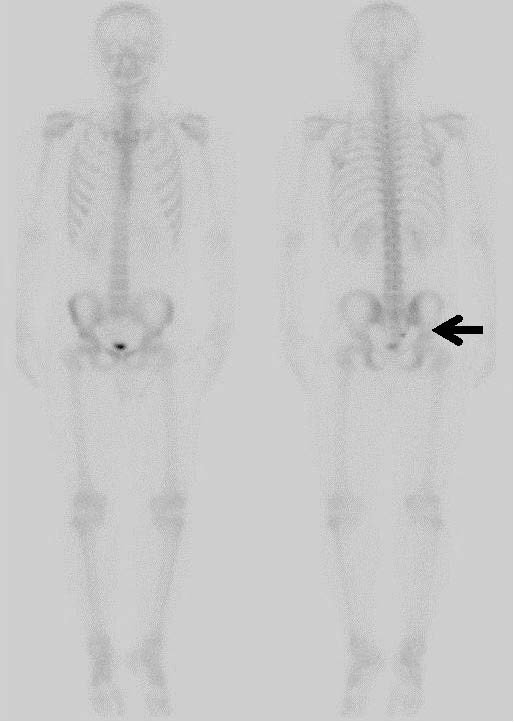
Bone scintigraphy. The tumor is depicted as an uptake in the right lower end of the sacrum (arrow).
